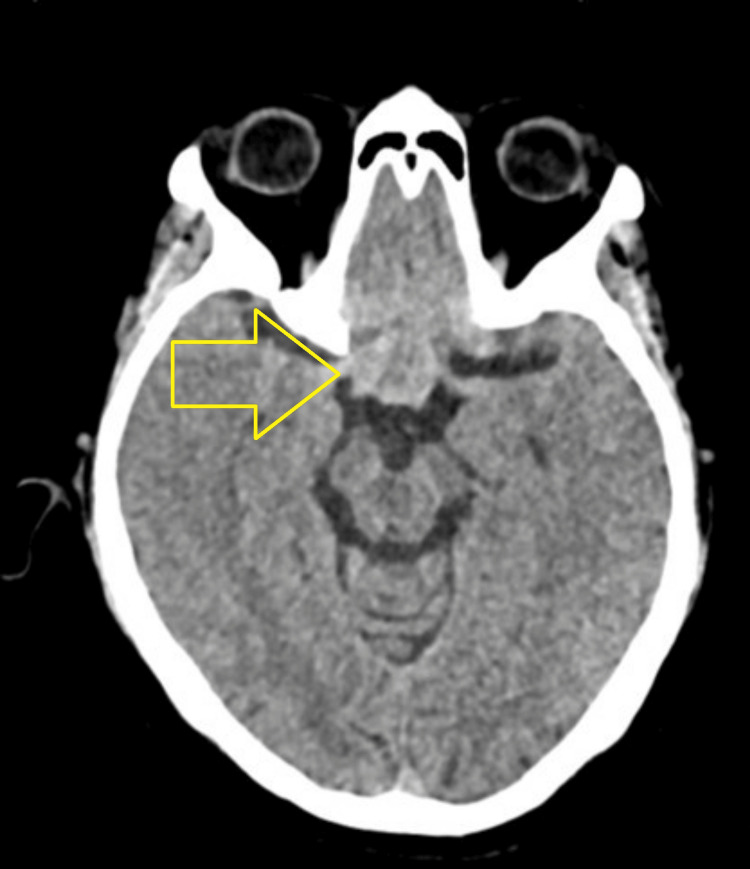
Non-contrast CT of the brain (axial image) showing a hyperdense suprasellar lesion (~65 Hounsfield units), suggestive of a thrombosed vascular lesion (arrow). CT: computed tomography
